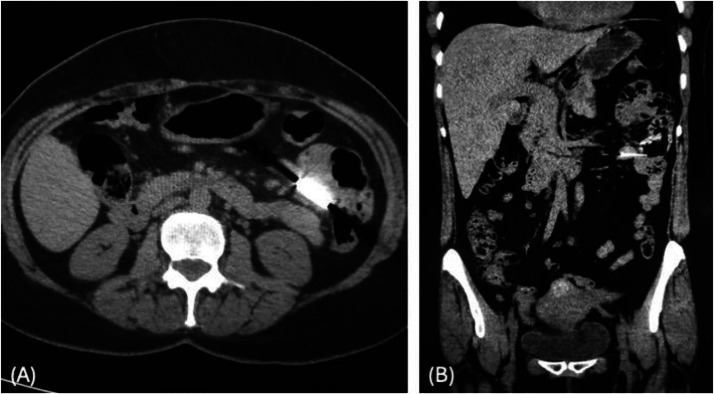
(A) Axial non-contrast CT of the abdomen showing a dense linear object within the small bowel in the left upper quadrant. (B) Coronal CT image confirming intraluminal location in the small intestine.
